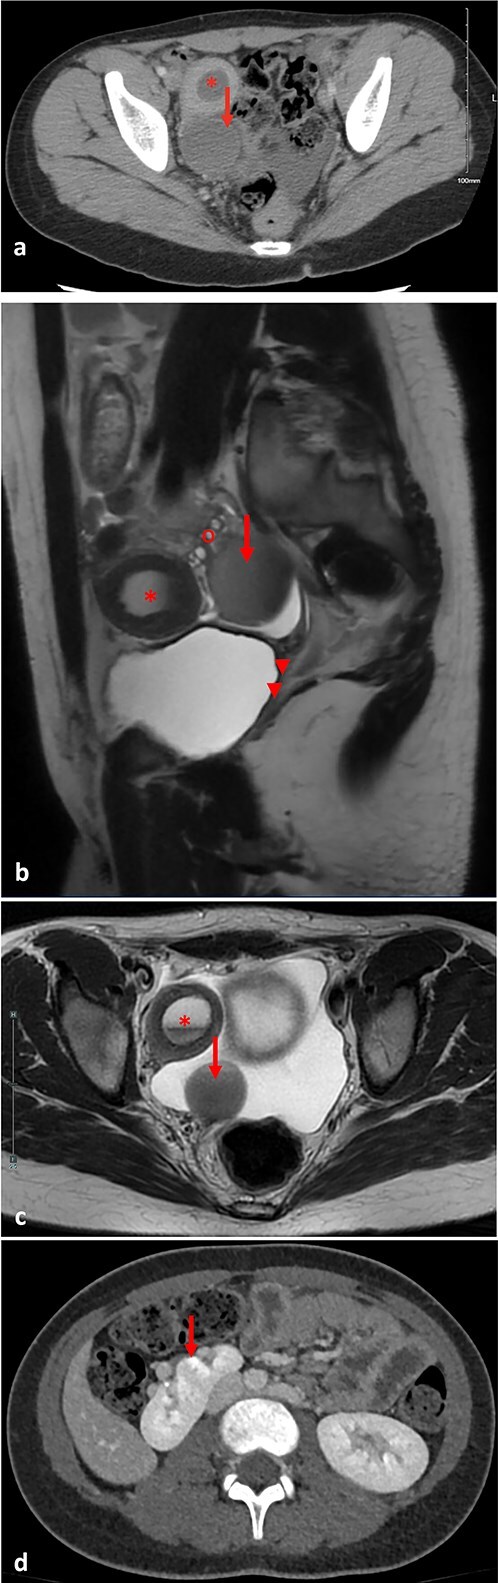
(a) Pre-operative axial computed tomography imaging, demonstrating a right uterine horn with hematometra (^*^) and a right adnexal cystic structure representing hematosalpinx (arrow). (b) Pre-operative pelvic parasagittal T2 weighted MRI, demonstrating a right uterine horn with hematometra (^*^), a right adnexal cystic structure representing hematosalpinx (arrow), normal right ovary (o), cervical aplasia, and vaginal atresia (arrowheads). (c) Pre-operative pelvic axial T2 weighted MRI, demonstrating a right uterine horn with hematometra (^*^), and a right adnexal cystic structure representing hematosalpinx (arrow). (d) Pre-operative abdominal axial T2 weighted MRI, demonstrating a right supernumerary kidney (arrow).
